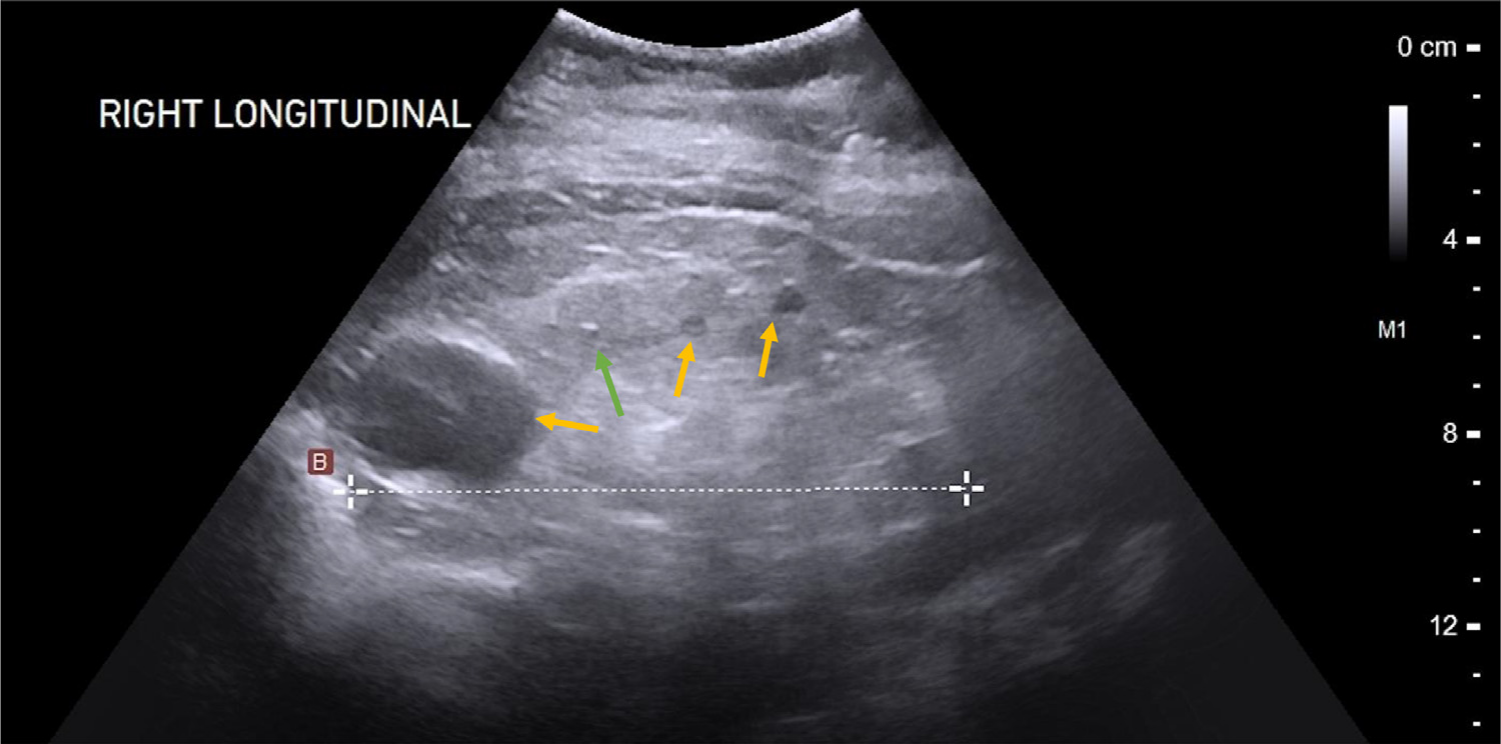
Point of care ultrasound performed in nephrology office of the right kidney. Multiple anechoic, well circumscribed cystic structures (orange arrows) and scattered echogenic foci (green arrow) are identified.
